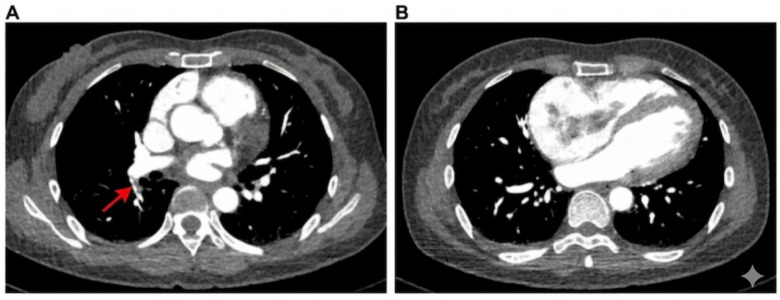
Total aortic computed tomographic angiography. (A) Pulmonary embolism in both lower lungs; (B) Right heart mass.
